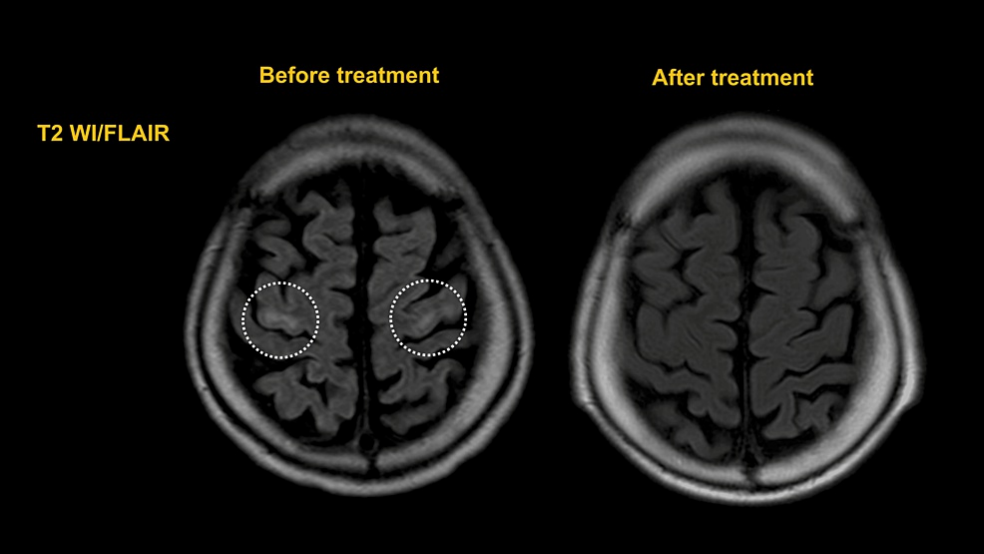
Head MRI (T2/WI FLAIR) Head MRI showing the changes that took place after treatment. T2 WI/FLAIR signals show high density of the precentral gyrus of the bilateral frontal lobes before treatment and its disappearance in the same area after treatment MRI: magnetic resonance imaging; T2 WI/FLAIR: T2-weighted/fluid-attenuated inversion recovery
